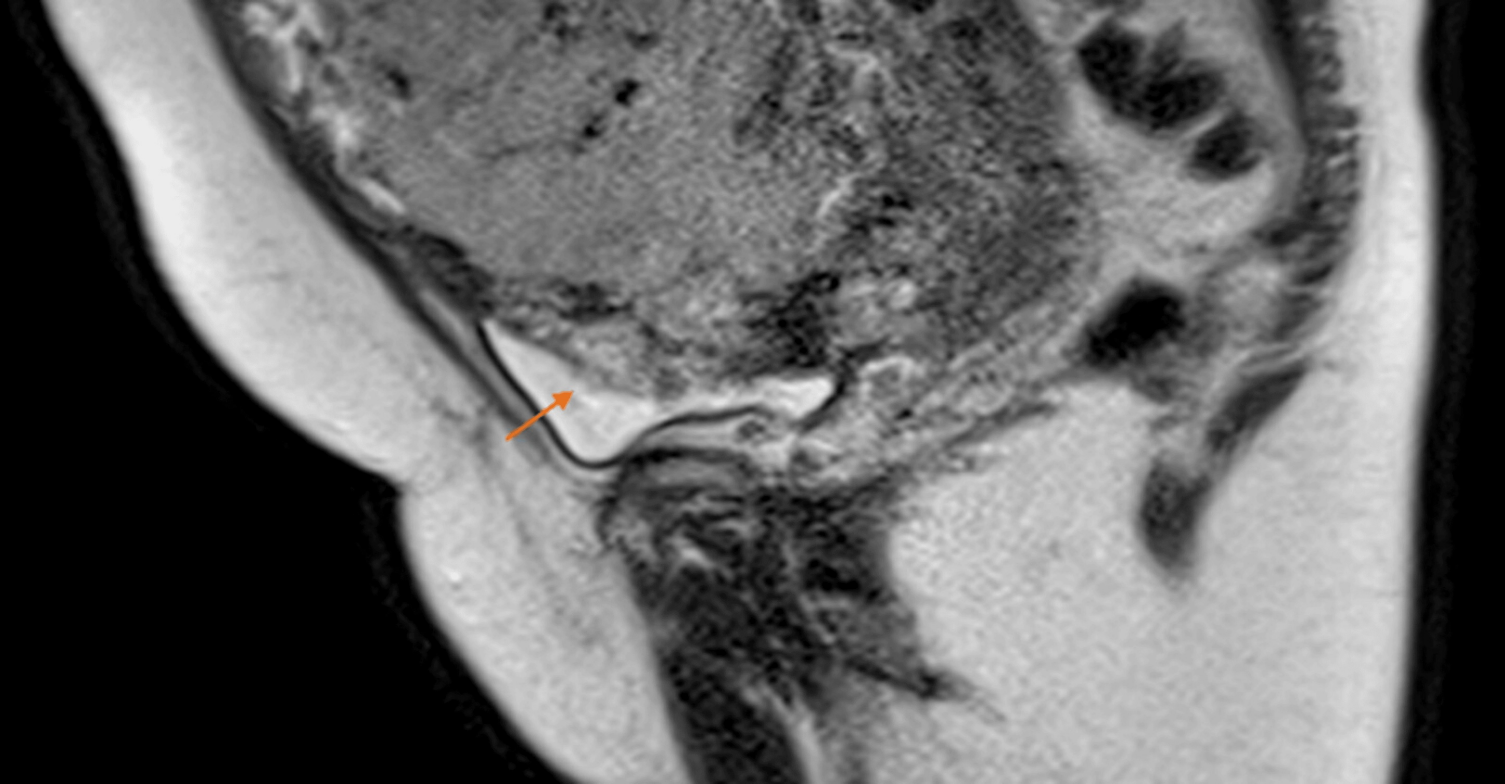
Magnetic resonance imaging in the sagittal plane in the T2 sequence without fat saturation extended towards the bladder. The loss of the hypointense line of the anterior bladder wall is observed secondary to the presence of a focal exophytic mass highly suggestive of placenta percreta.
